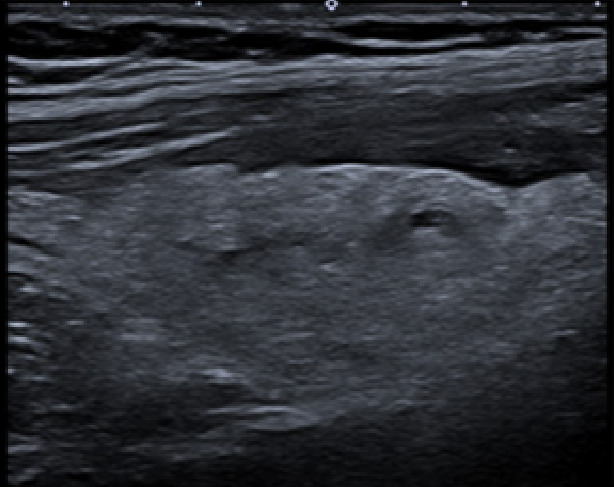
Radiology-performed ultrasound of the right iliac fossa with a high frequency linear probe demonstrating significant hyperinflammatory changes. When taking clinical presentation into account, this report concluded that appearances were consistent with likely appendicitis with perforation.
